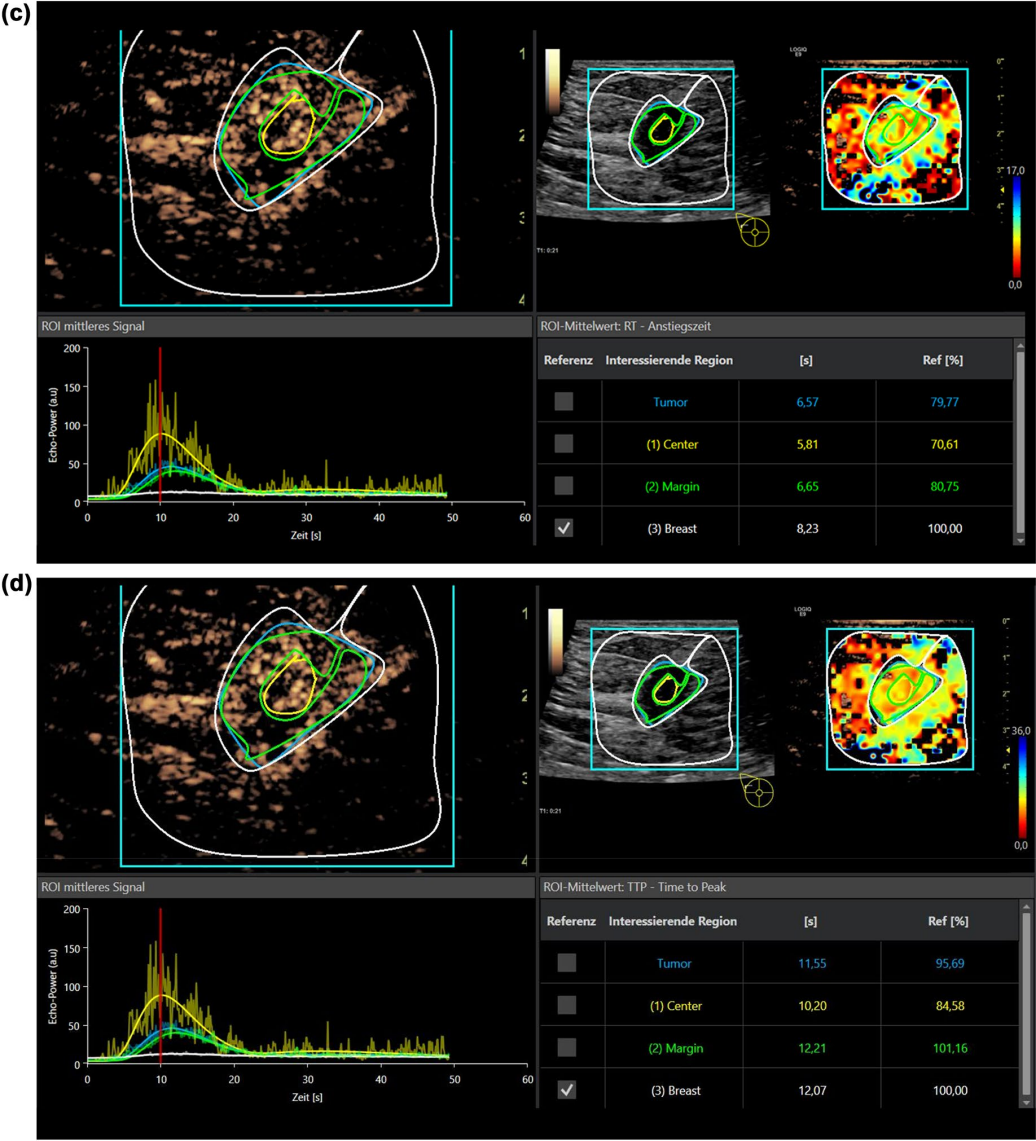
(a) Evaluation of dynamic CEUS micro-vascularization of a malignant breast lesion after i.v. application of 2.4 ml ultrasound contrast media using external perfusion software VueBox. The VueBox screen is divided into four sections. Up left, the original CEUS image with the manually drawn Regions of Interest (ROI). Up right, the perfusion imaging with false colors showing hyperperfusion in red shades. Down left, the Time Intensity Curve analysis (TIC) of the Regions of Interest and down right the numeric values of the regions. The healthy breast tissue is taken as reference. Each perfusion parameter is calculated in an extra step. Case of a small malignant breast tumor. Contrast enhanced evaluation up to 60 s for PE. In parametric imaging irregular red and yellow false colors for tumor micro-vascularization, blue for the surrounding tissue. High and fast wash in and rapid wash out in time intensity curve analysis (TIC) as criteria for malignancy. (b) Same case of a malignant breast lesion with evaluation of wash in area under the curve (AUC). (c) Same case of a malignant breast lesion with evaluation of Rise Time (RT). Less differences are seen in parametric imaging compared to the surrounding tissue. (d) Same case of a malignant breast lesion with evaluation of Time to Peak (TTP), with hardly any differences in parametric imaging compared to the surrounding tissue.
